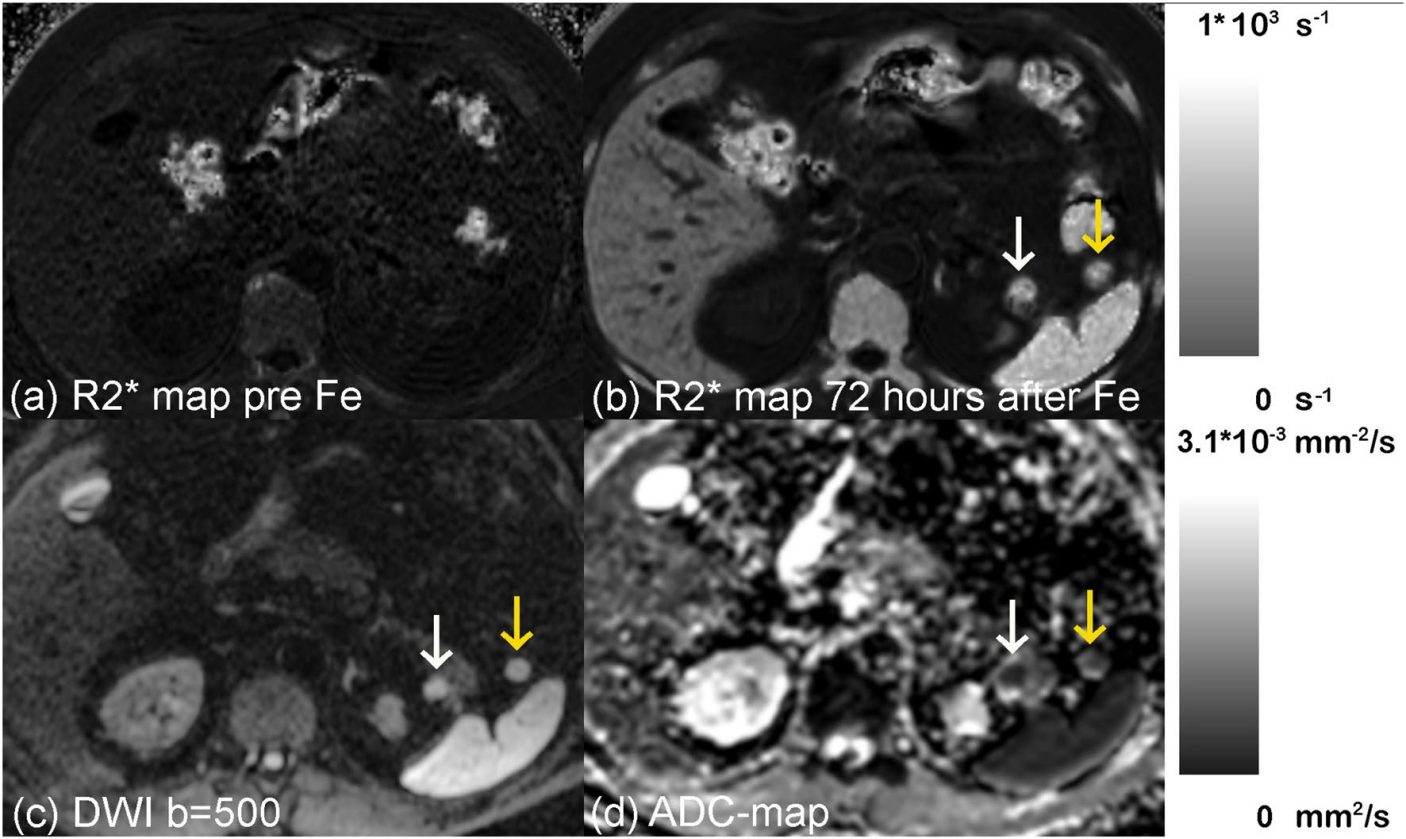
R2* mapping and DWI in a presumed splenule (pt. 2). Pre-contrast R2* maps demonstrate low R2* in the upper abdominal organs (a). After administration of ferumoxytol, the USPIO particles are phagocytosed by the MPS. 72 h after ferumoxytol administration, the intrapancreatic splenule (white arrow), a splenule in the hilus of the spleen (yellow arrow), the liver, and the spleen all show elevated R2* values (b). With DWI, the splenules have very similar signal intensity to the spleen on b = 500 s/mm2 (c) and similar ADC values as the spleen before ferumoxytol administration (d)
